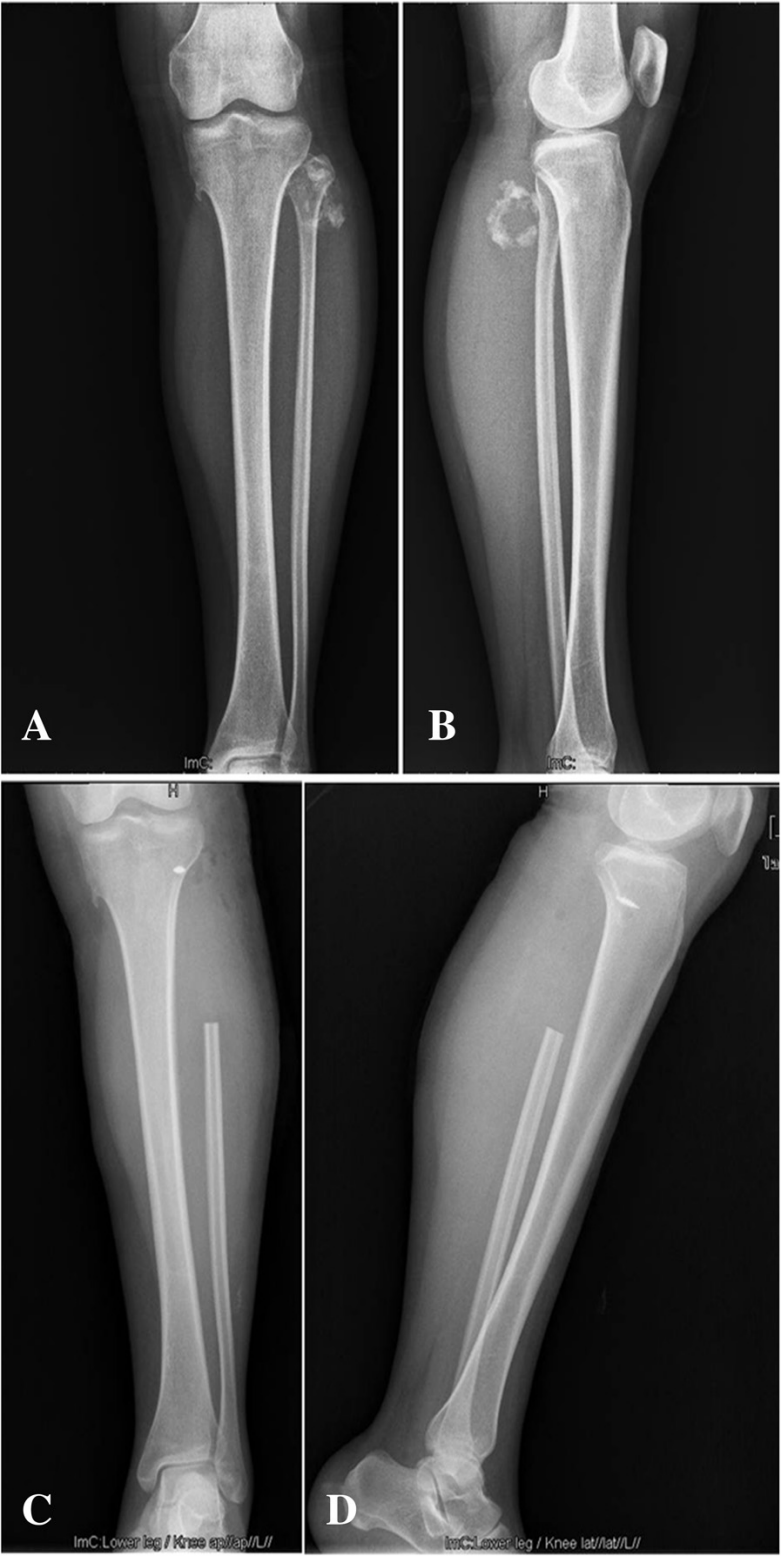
Radiographs of the patient’s left shank. A, B Anteroposterior and lateral views show a bone tumor with a ‘popcorn’ and ‘smoke ring’ pattern of calcification on the posterolateral aspect of the left proximal fibula, respectively. C, D Postoperative radiographs show the absence of the left proximal fibula and an anchor inserted the lateral tibial metaphysis.
